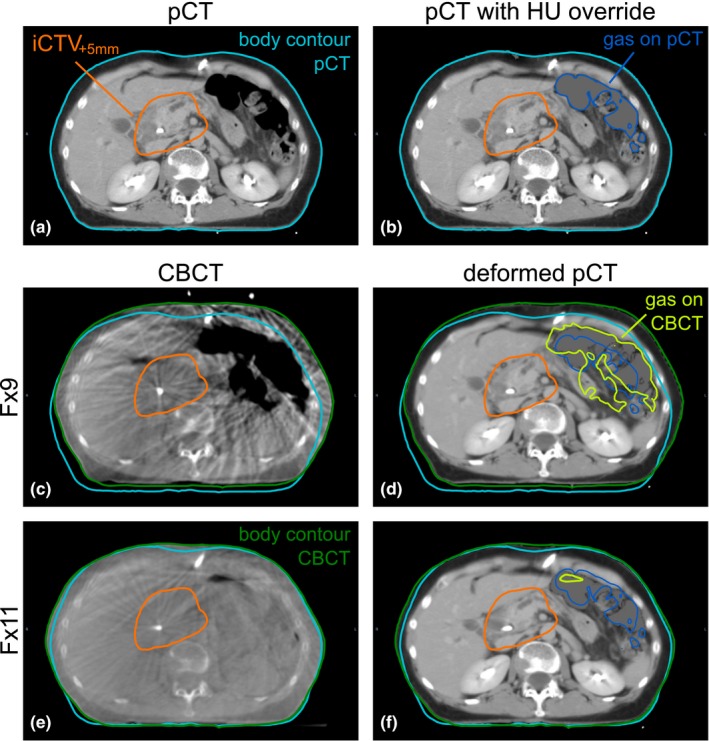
Anatomical changes. Example of anatomical changes in patient 8: (a) pCT, (b) pCT with HU override of 0 for gastrointestinal gas, (c) CBCT for fraction 9, (d) deformed CT for fraction 9, (e) CBCT for fraction 11, and (f) deformed CT for fraction 11. Images in (d and f) are for the fiducial marker‐based rigid registration to the pCT. Contours are shown for iCTV
